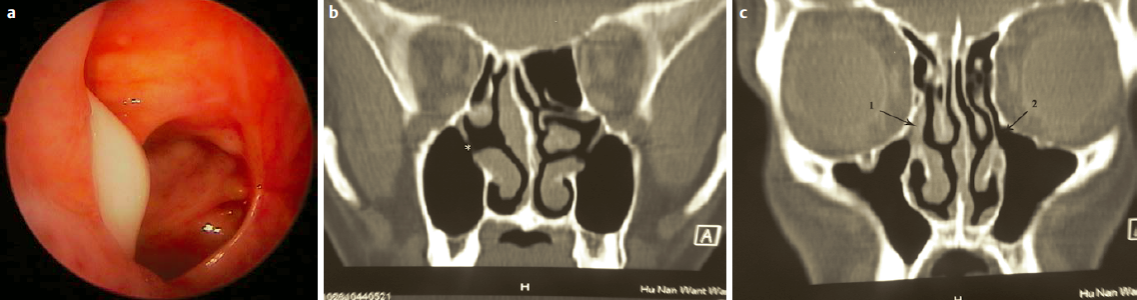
Missed ostium sequence. a) Typical secretion drop directly behind obvious remnants of the uncinate process in MOS of the right side after previous surgery. b) In the coronal CT scan a larger opening of the maxillary sinus is seen in the posterior part of the middle meatus (*). c) In the area of the natural ostium, however, remnants of the uncinate process and soft tissue are revealed (mucosal swelling, scars (=1) with obstruction of the natural ostium in contrast to free drainage on the left side (=2)).
